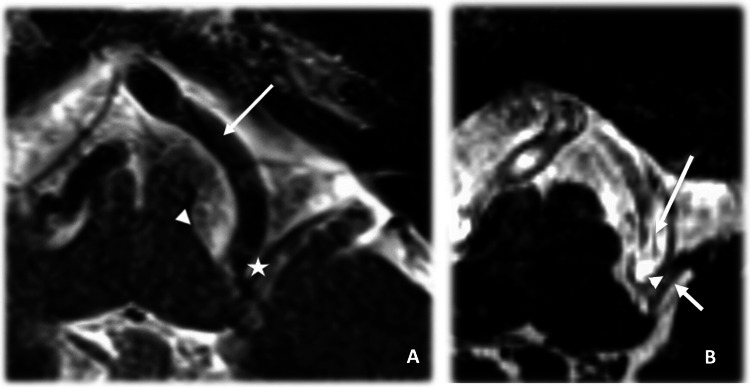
A Left axial T2 MR image at the medullary level demonstrating the vertebral artery (VB, large arrow) anterior to the root entry zone of the glossopharyngeal nerve (IX star), indicating a neurovascular conflict. The olivary grove is indicated by the arrow head; B Axial 3D CISS (T2) MR image at the level of the medulla demonstrating the vertebral artery exerting a neurovascular conflict to the glossopharyngeal nerve (IX, arrowhead). The posterior inferior cerebellar artery (PICA, small arrow) is also seen in contact with the IX nerve, suggesting an additional potential conflict
